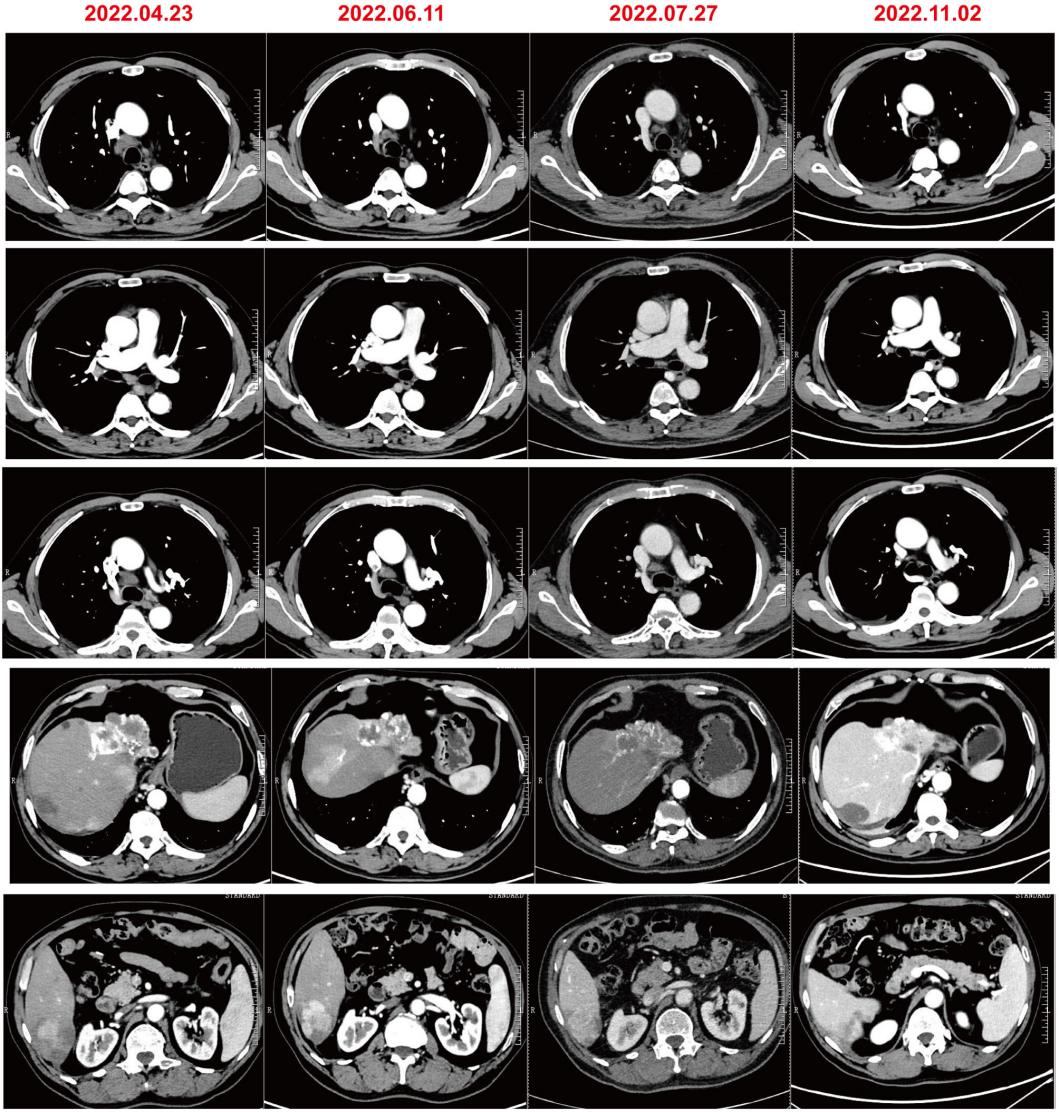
Evaluation of radiographic response evaluation.
